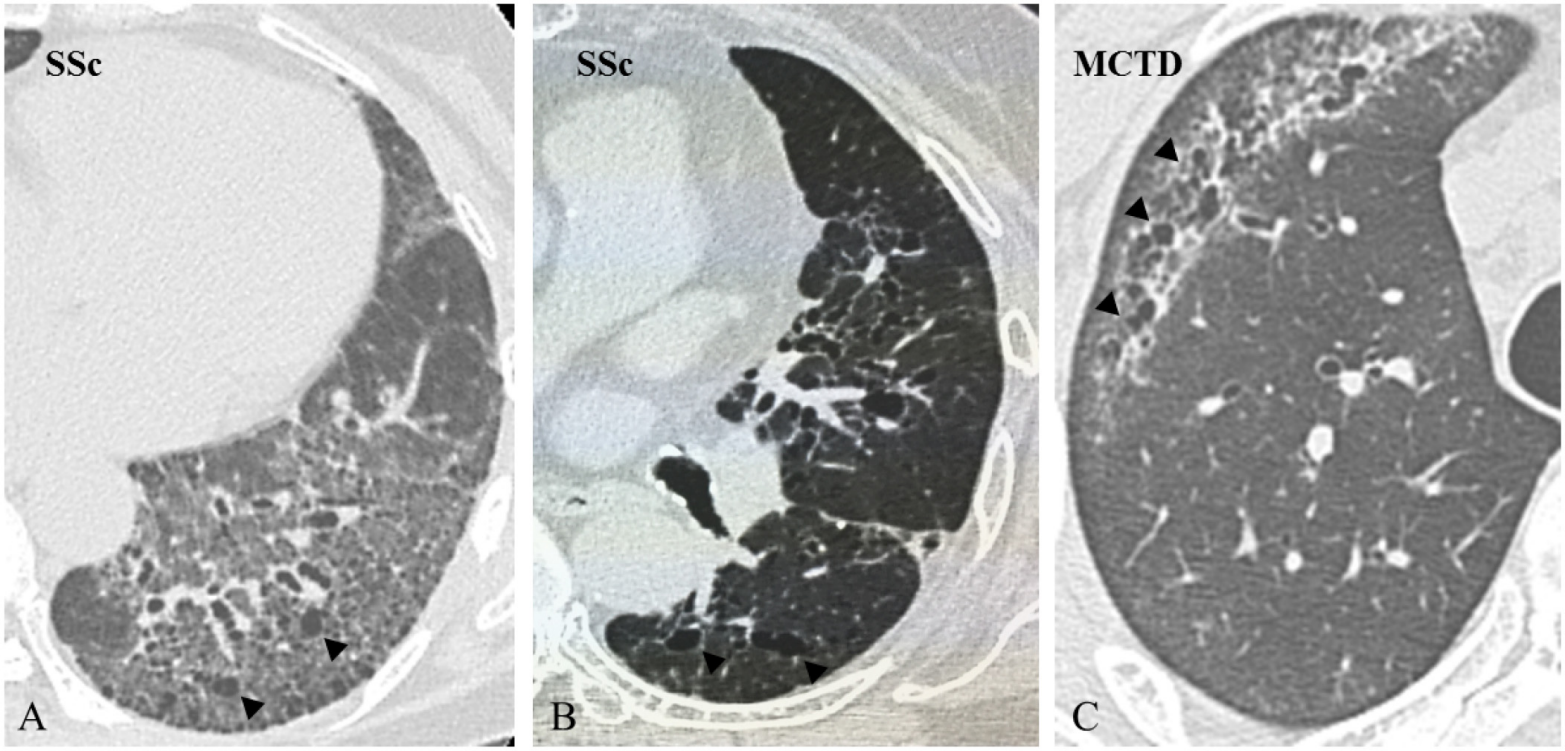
HRCT scans of cyst formation. (A-B) HRCT scan demonstrates cyst formation (arrowheads) with pulmonary fibrosis, traction bronchiectasis, and architectural distortion in two patients with SSc-ILD. There is no continuity between the cysts and traction bronchiectasis. (C) HRCT scan shows cysts (arrowheads) with opacity separated from the pleura in a patient with MCTD-ILD.
